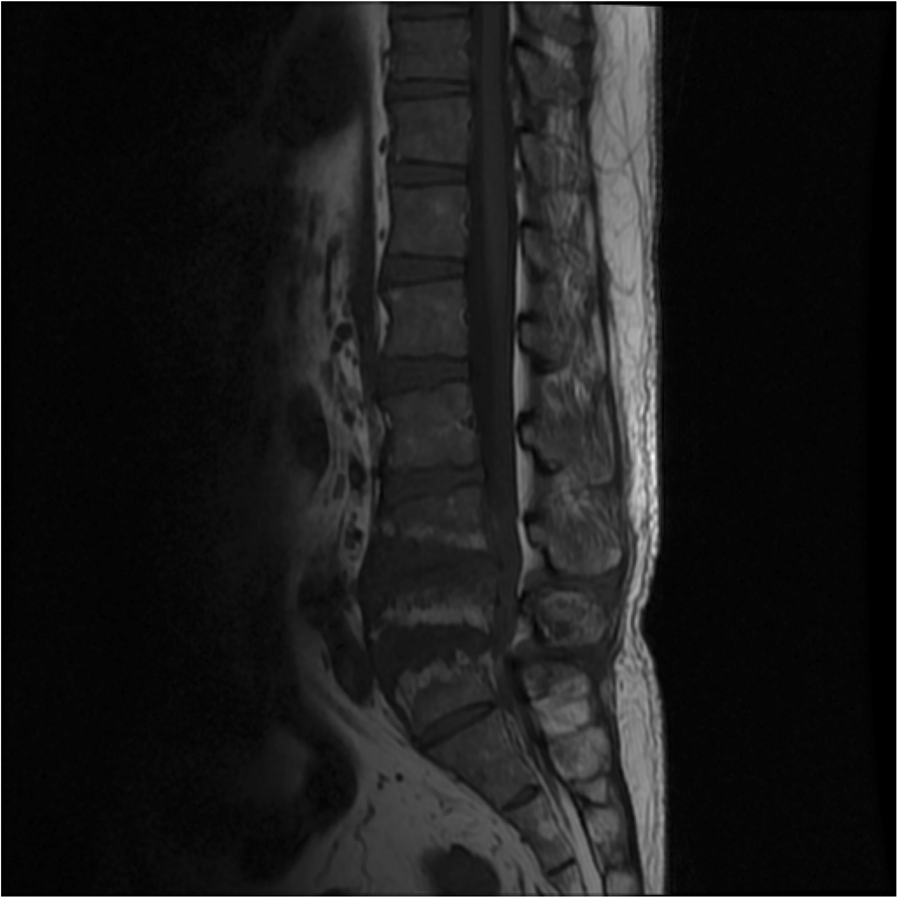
Irregular endplate erosion manifested as hypointensity on T1-weighted imaging
